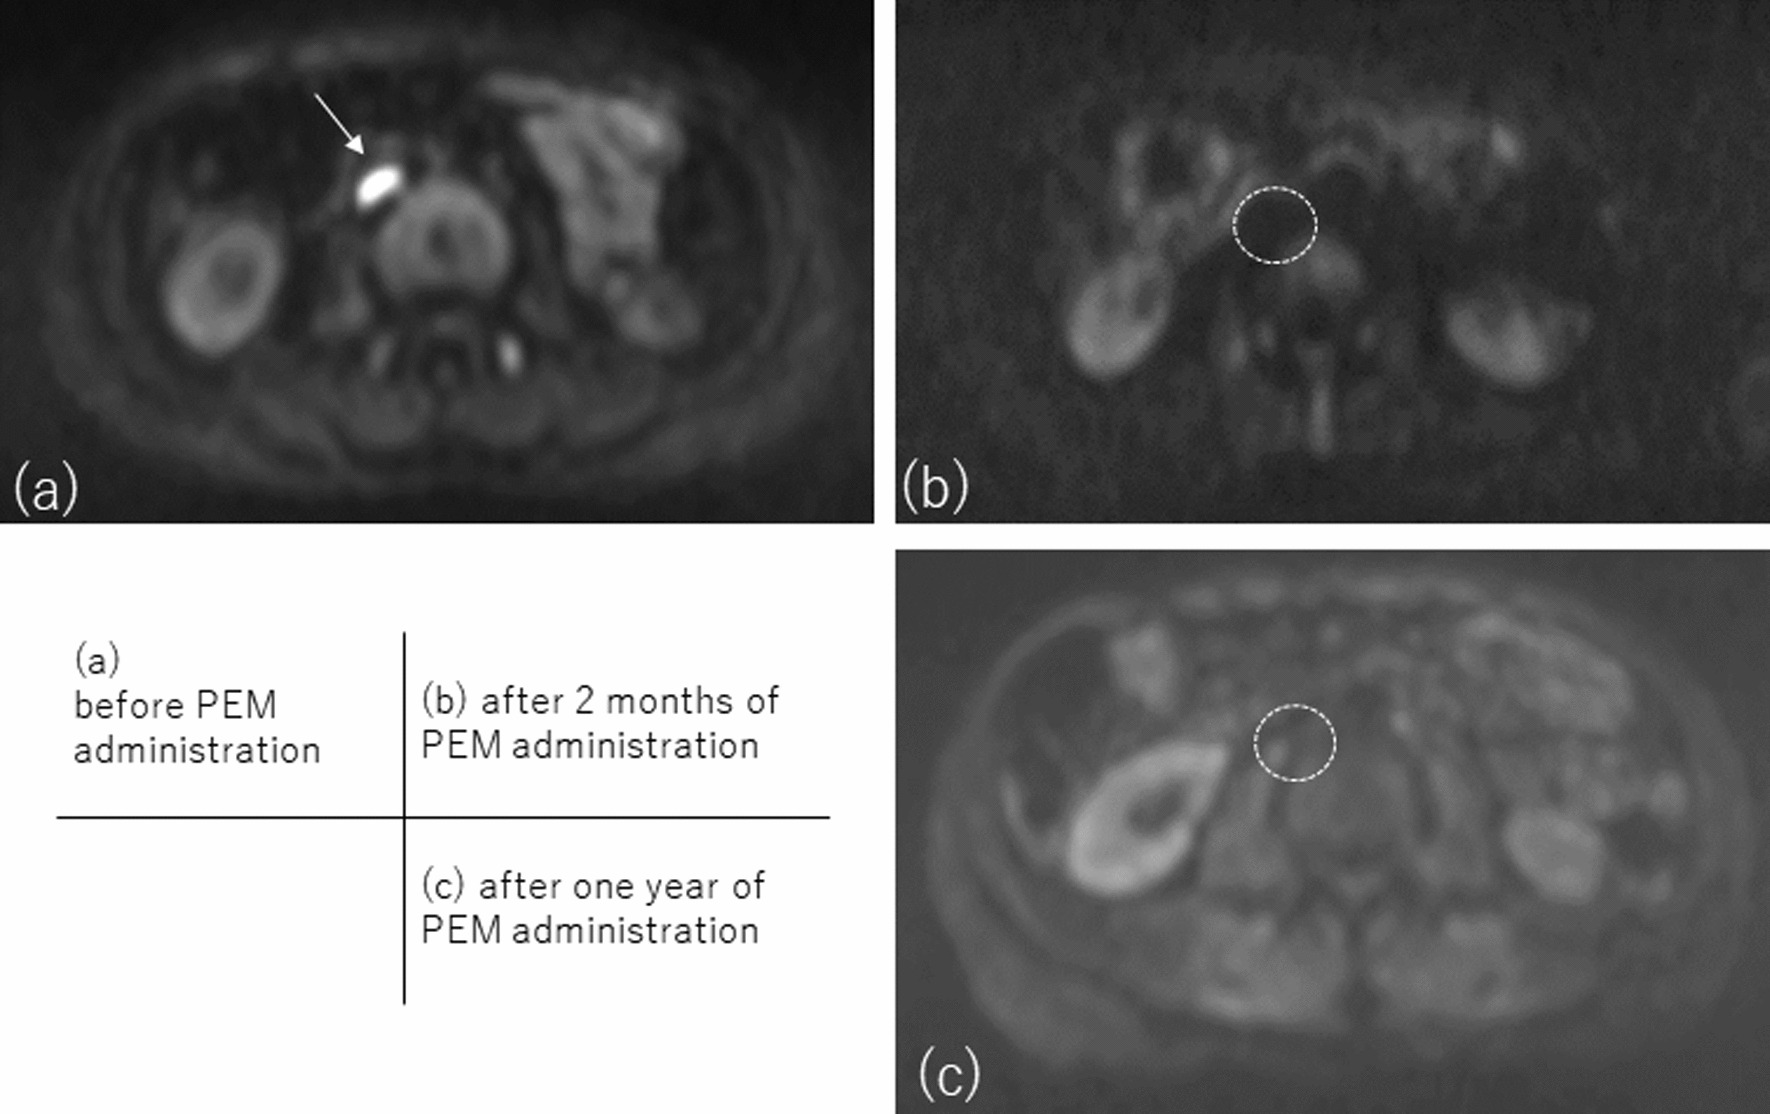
Progress of PEM treatment of para-aortic lymph node metastasis of breast cancer, using deffusion weighted MRI: a before PEM administration, the para-aortic lymph nodes were metastatic (arrow). b after 2 months of PEM administration, para-aortic lymph nodes in the circular area were disappeared. c After one year of PEM administration, the para-aortic lymph nodes in the circular area remain obliterated
